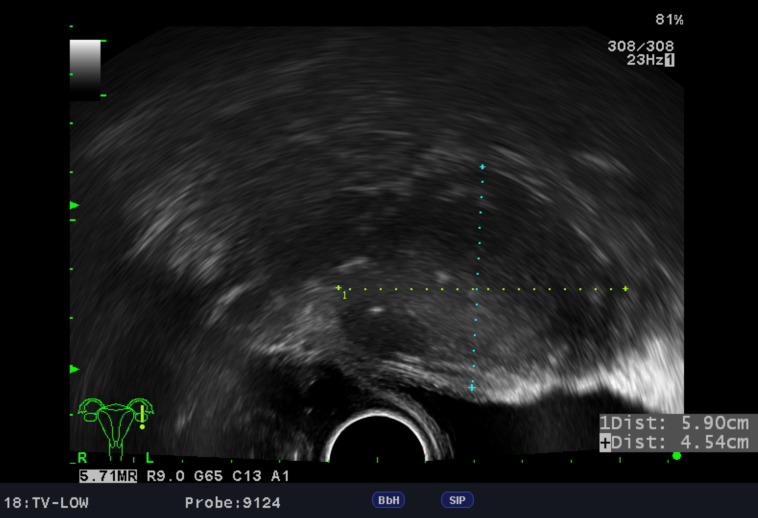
Transvaginal ultrasound of the left adnexal tumor (sagittal view). 1: Longitudinal diameter, +: Transverse diameter.
